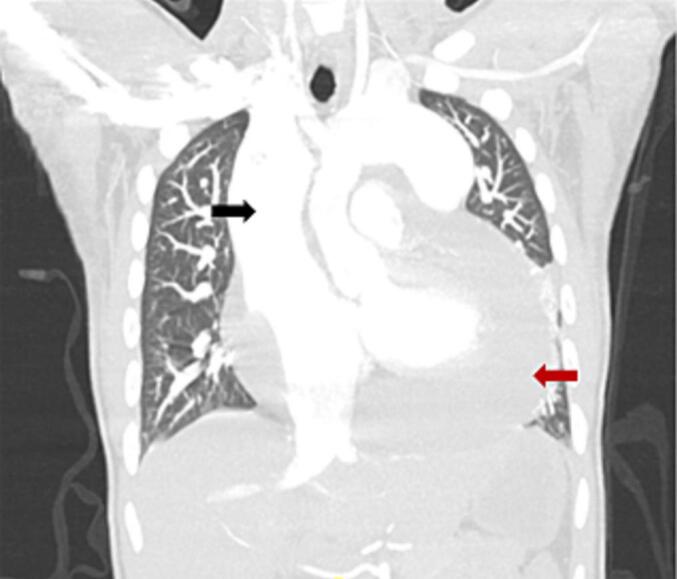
Computed tomography angiogram of the chest with IV contrast before initial pericardiocentesis. This figure shows computed tomography angiogram of the chest obtained upon presentation before the initial pericardiocentesis, revealing a large pericardial effusion (red arrow), dilated superior vena cava (SVC) (black arrow).
